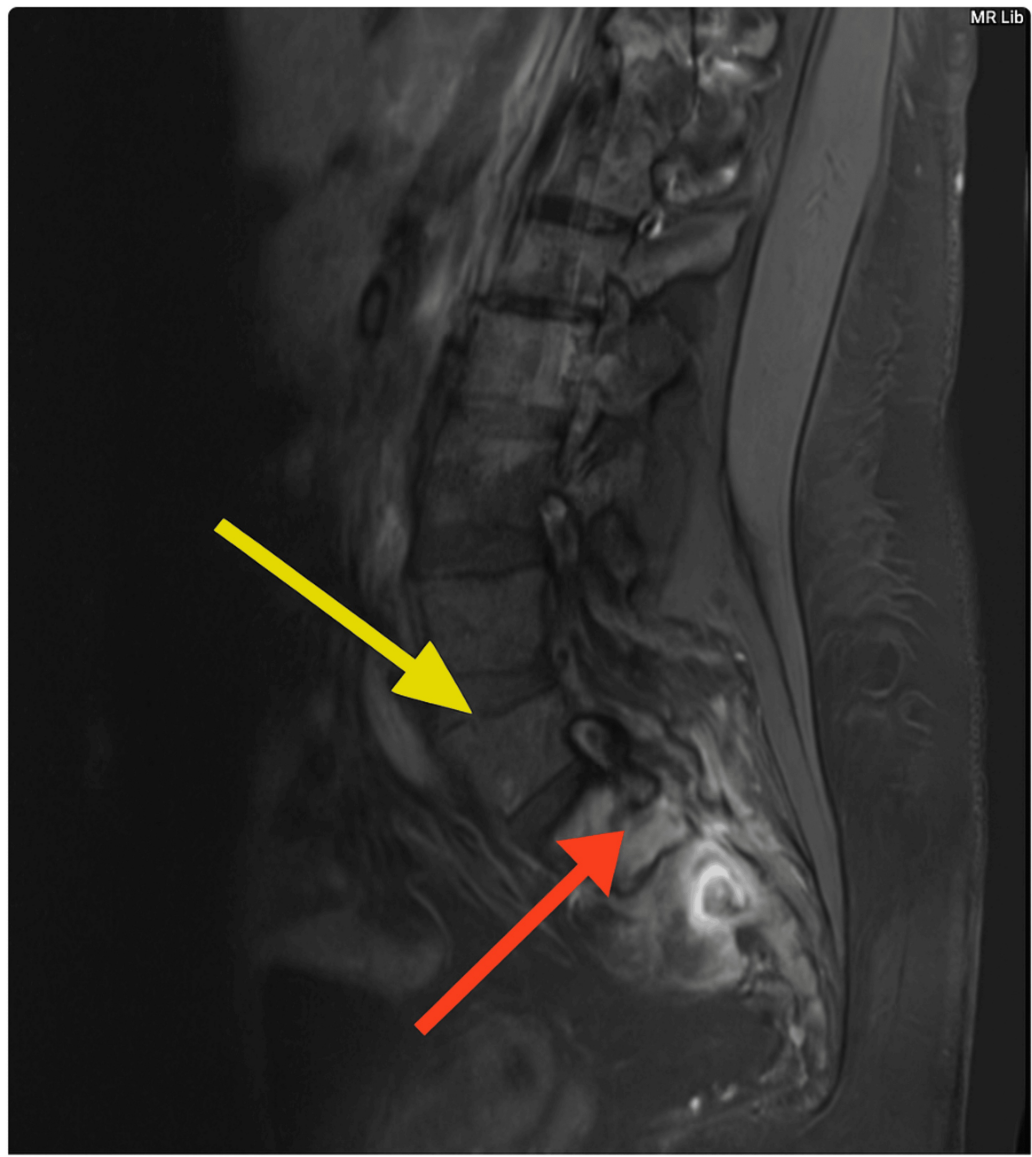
MRI without contrast revealed erosion of the S1 spinous processes (yellow arrow) and fluid collection adjacent to inflammatory changes consistent with abscess formation (red arrow) and osteomyelitis.
